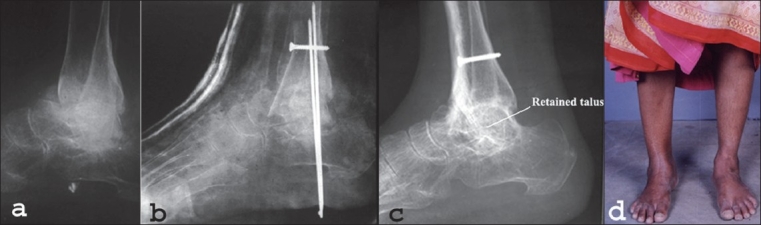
Lateral X-ray (a) of ankle and foot in a 58-year-old woman with eight months' old neglected avascular necrosis of the talus. Immediate postoperative lateral X-ray (b) of the same shows tibiotalar arthrodesis after removal of loose pieces from necrotic talar body and anterior sliding graft from tibial stabilized with transcalcaneal k-wires. Lateral X-ray (c) of ankle of the same patient at 3 years follow-up shows incorporation of tibial and body of talus with cross trabeculation. The clinical photograph (d) at 3 years follow-up shows normal looking appearance
